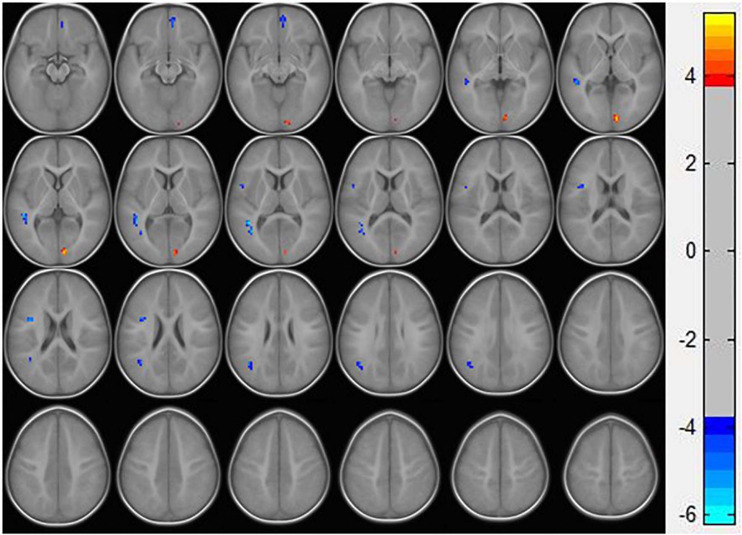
Statistically significant differences between ASD boys and TDs are shown in a ReHo map of the whole brain with MRI. The ASD boys showed a significant ReHo increase in the right calcarine (warm colors) as well as a decrease in the opercular part of the left inferior frontal gyrus, left middle temporal gyrus, left angular gyrus, and right medial orbital frontal cortex with decreased ReHo values (cold colors). A T-score bar is shown on the right. Warm and cold colors denote increases and decreases in ReHo, respectively.
